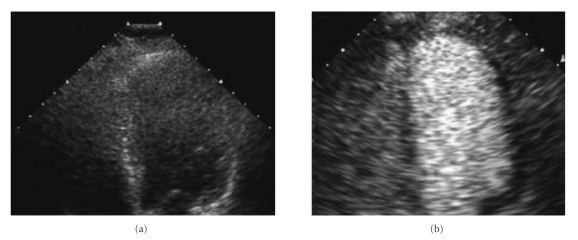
(a) Apical 4-chamber view (left ventricle on right, end-diastole) with harmonic imaging. (b) Same view with enhancement due to intravenous microbubble contrast agent. Image shows marked improvement of endocardial border definition, especially at apex and in mid segment of anterolateral wall.
